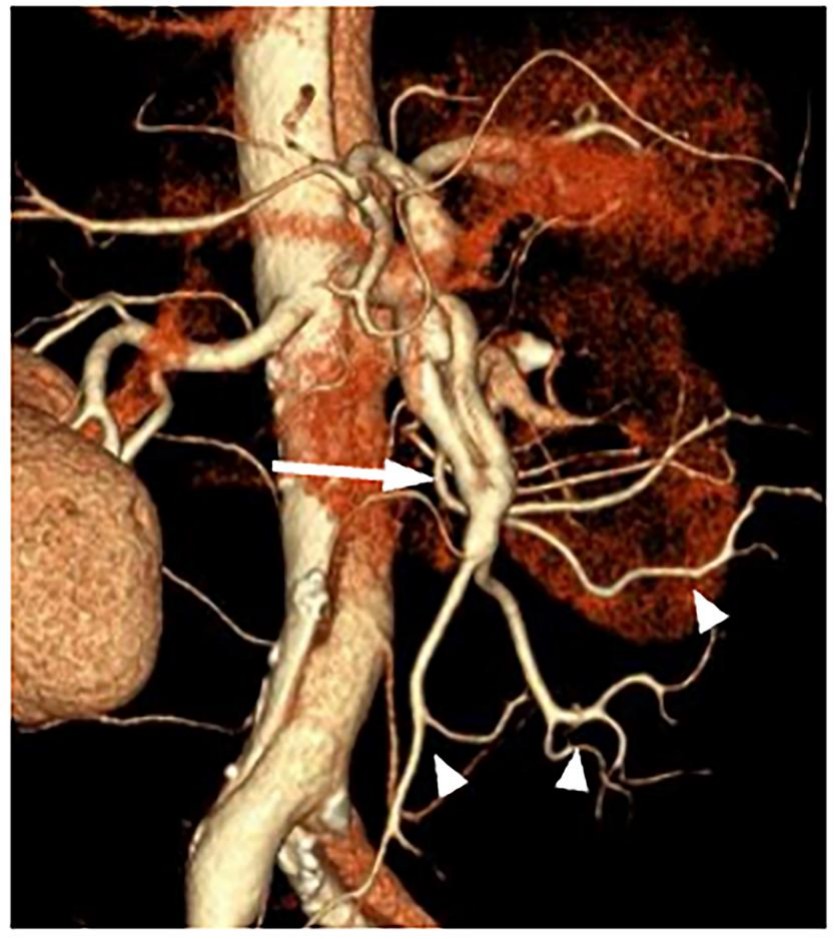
3D-CT findings 10 years after superior mesenteric artery plasty. The site of fenestration (white arrow) has well-visualized peripheral vasculature (white arrowheads), indicating long-term patency of the reconstructed SMA.
